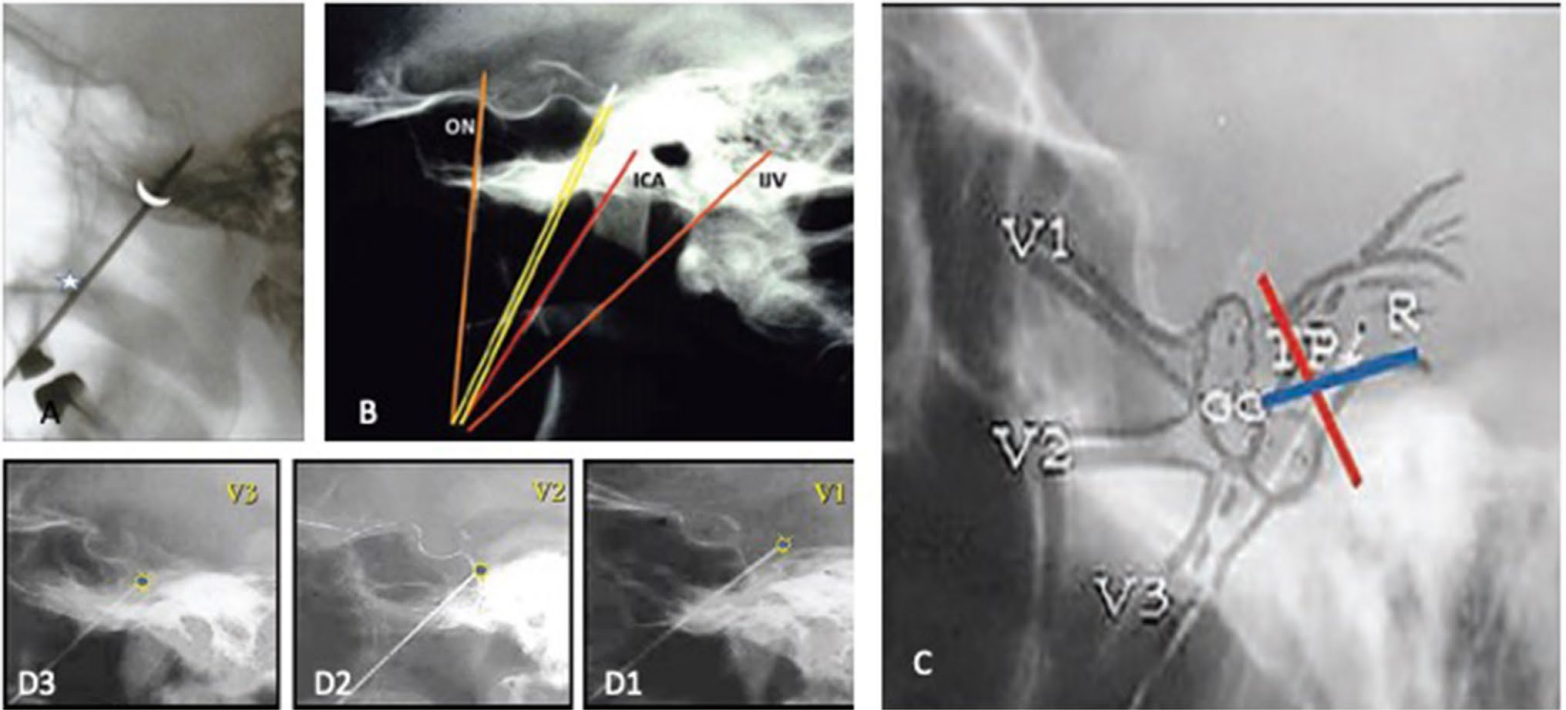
Fluoroscopic control of the position of the needle. Imaging control should be performed at each step of the procedure. A strict lateral view is essential (this is checked by aligning the acoustic meati). A The FO is marked by the demilune. An important radiological landmarking the face is the posterior angle of the maxillary sinus (white star). The needle should draw a line between these two points. The tip of the needle should be placed in the triangular plexus. The landmark is the intersection of the clivus and the upper petrous ridge. B False trajectories. An excessive postero-lateral direction could puncture the internal jugular vein (IJV) at jugular foramen or the internal carotid artery at entrance into the petrous carotid canal. An excessive medial direction could enter the foramen lacerum and injure the internal carotid artery (ICA) at its C5 segment. An excessive anterior direction could penetrate the orbital apex through the inferior orbital fissure and injure the optic nerve (ON). The appropriate trajectory is yellow. C Projection of the peripheral (V1, V2 and V3) branches, ganglion (GG), and trigeminal plexus (TP) on a lateral skull X-Ray. The triangular plexus is situated at the intersection of the projection of the clivus and upper petrous ridge. D Respective projections for the probe for V1-V2-V3 lesions
